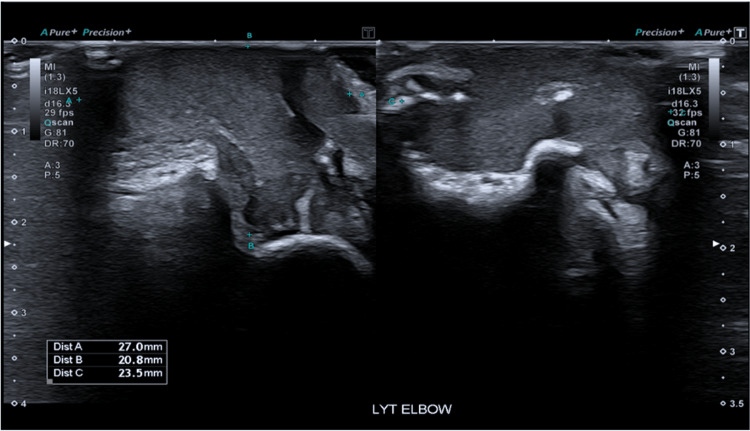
Ultrasound Left Elbow: Persistent 27mm x 21mm x 23mm superficial collection adjacent to and eroding into the left olecranon with marked surrounding inflammatory change. No elbow joint effusion.
